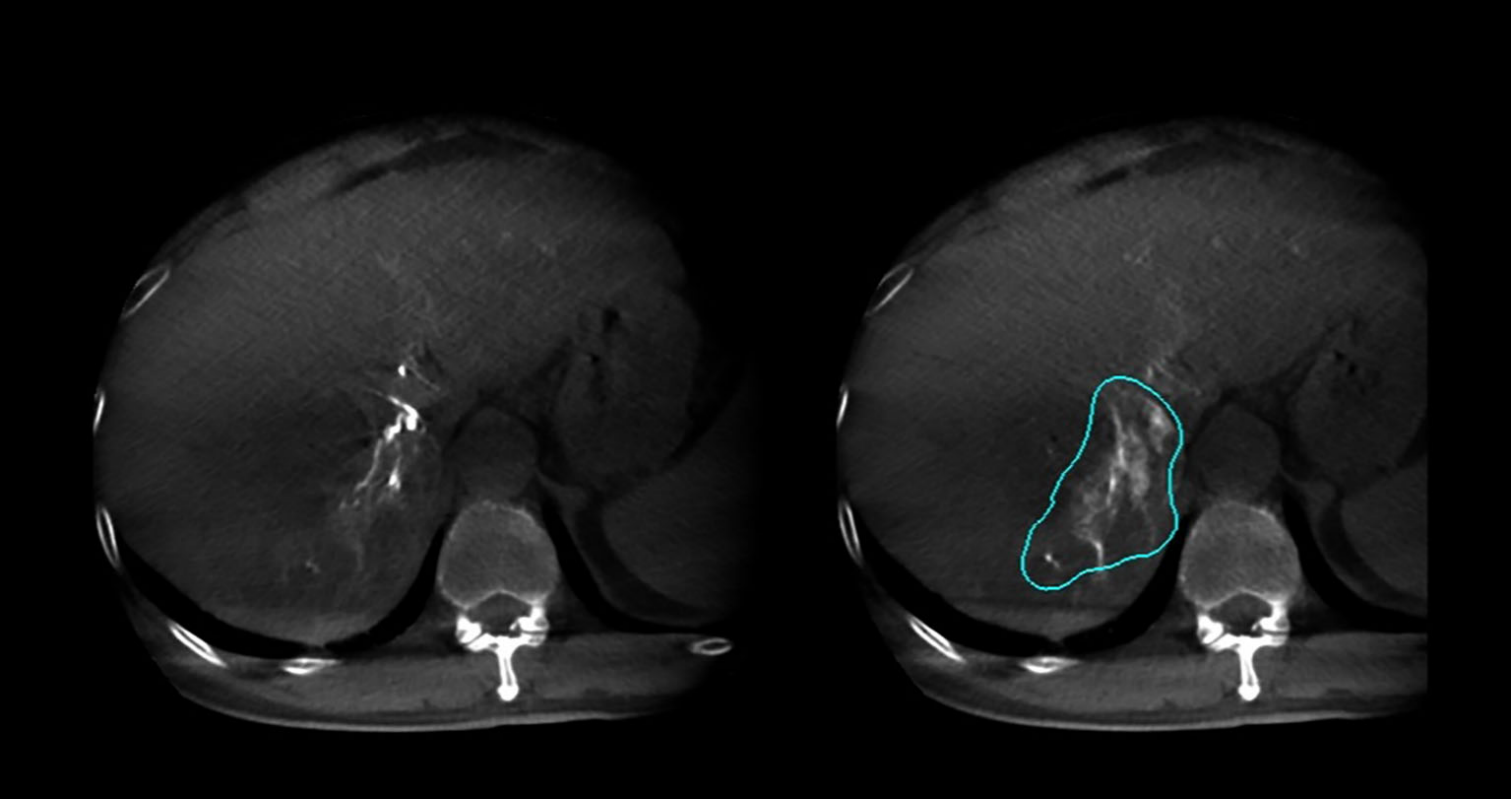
Dual CBCT images from the same cross-section were used to create a three-dimensional reconstruction model of the tumor.
